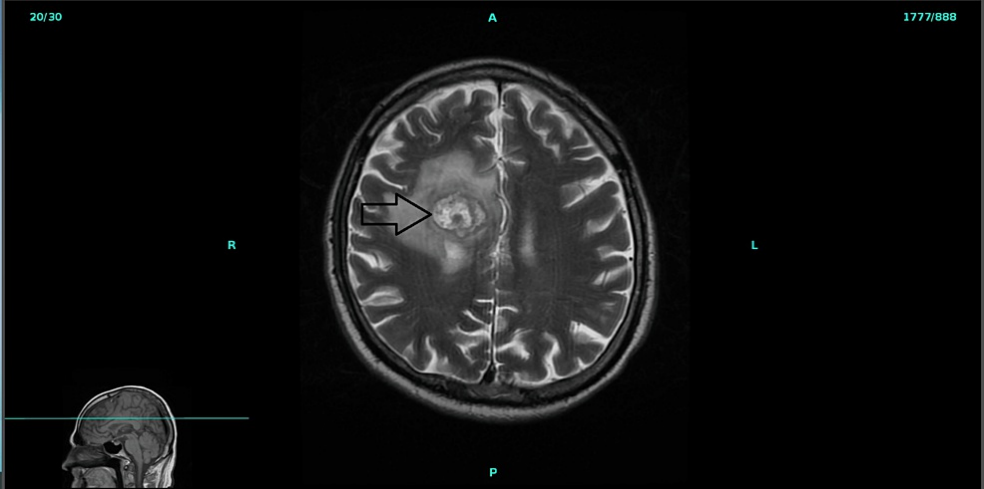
MRI brain showing a 3.4 cm ring-enhancing lesion in the medial aspect of the right parietal lobe.
